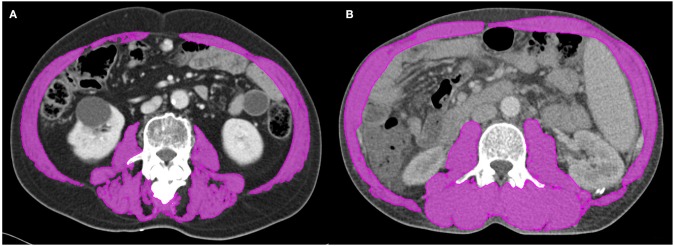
Segmented computed tomography (CT) images of patients with (A) and without (B) sarcopenia who had similar body mass indices. Skeletal muscle was measured using the MIM Vista software (MIM corp., version 6.1, OH, USA).
